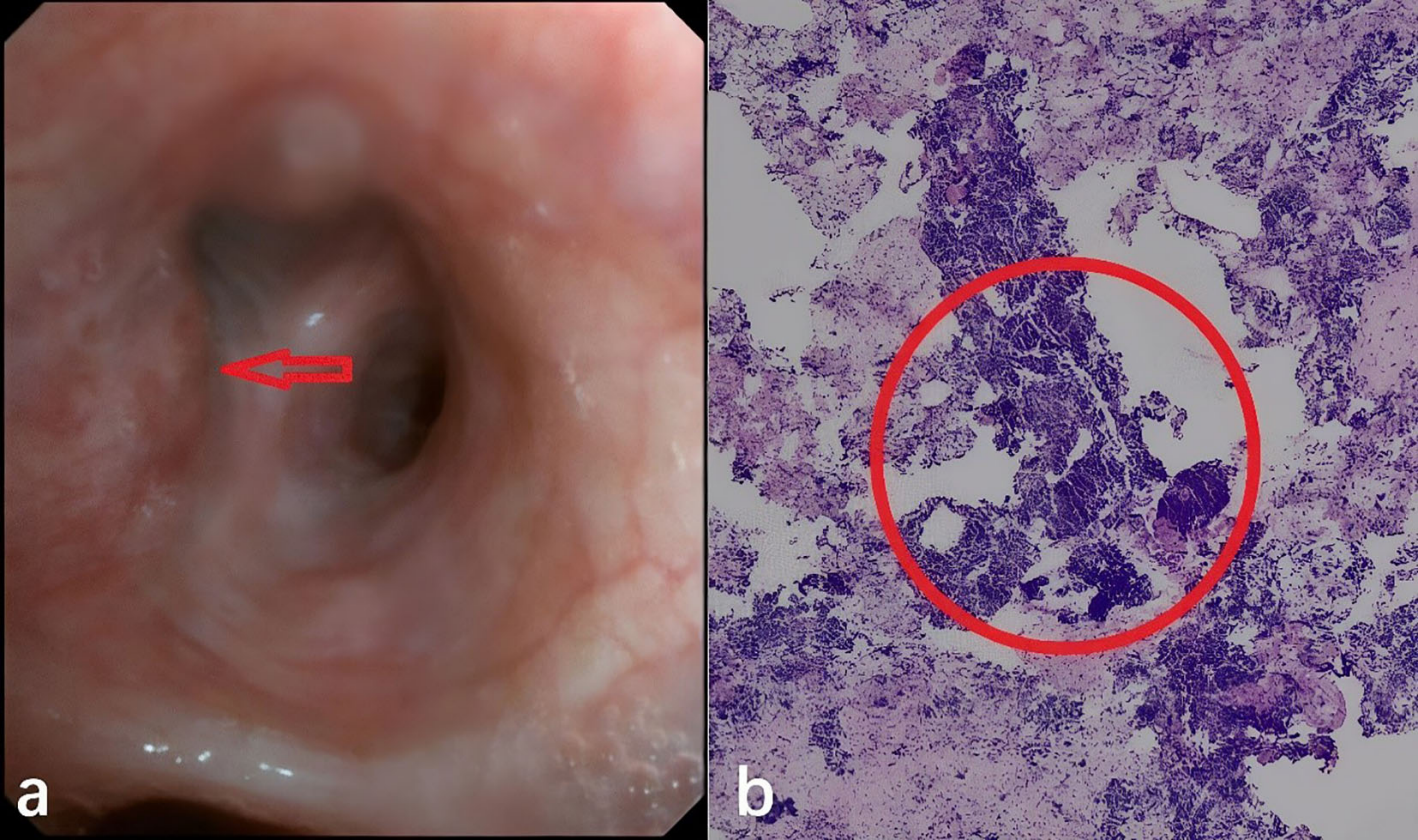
(a) Patient’s tracheoscopy picture at the trachea opening in the upper lobe of the left lung, the arrow indicates tumor invasion into the mucosal layer of the bronchus. (b) Patient’s pathological slides of seven groups of lymph node puncture biopsies performed by ultrasound tracheoscopy. Within the red circles, it’s shown that small, round, nest-like, and hyperchromatic neuroendocrine tumor cells.
